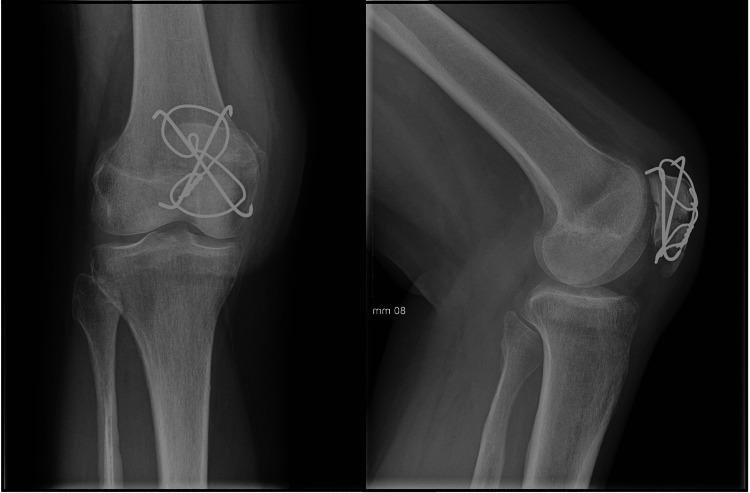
Anteroposterior and lateral X-rays of a healed patellar fractures four months after fixation with the modified crossed pin tension band technique.
